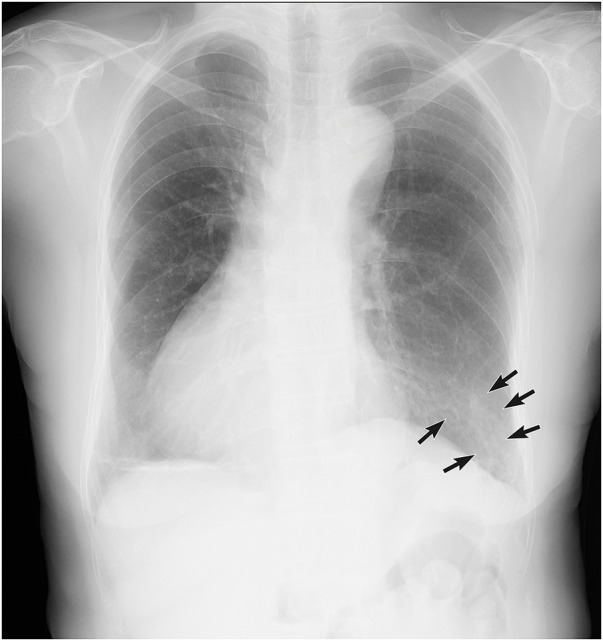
The chest X-ray shows a cord-like shadow in the left lower lung field, lateral region, continuous with a blood vessel (black arrows).
